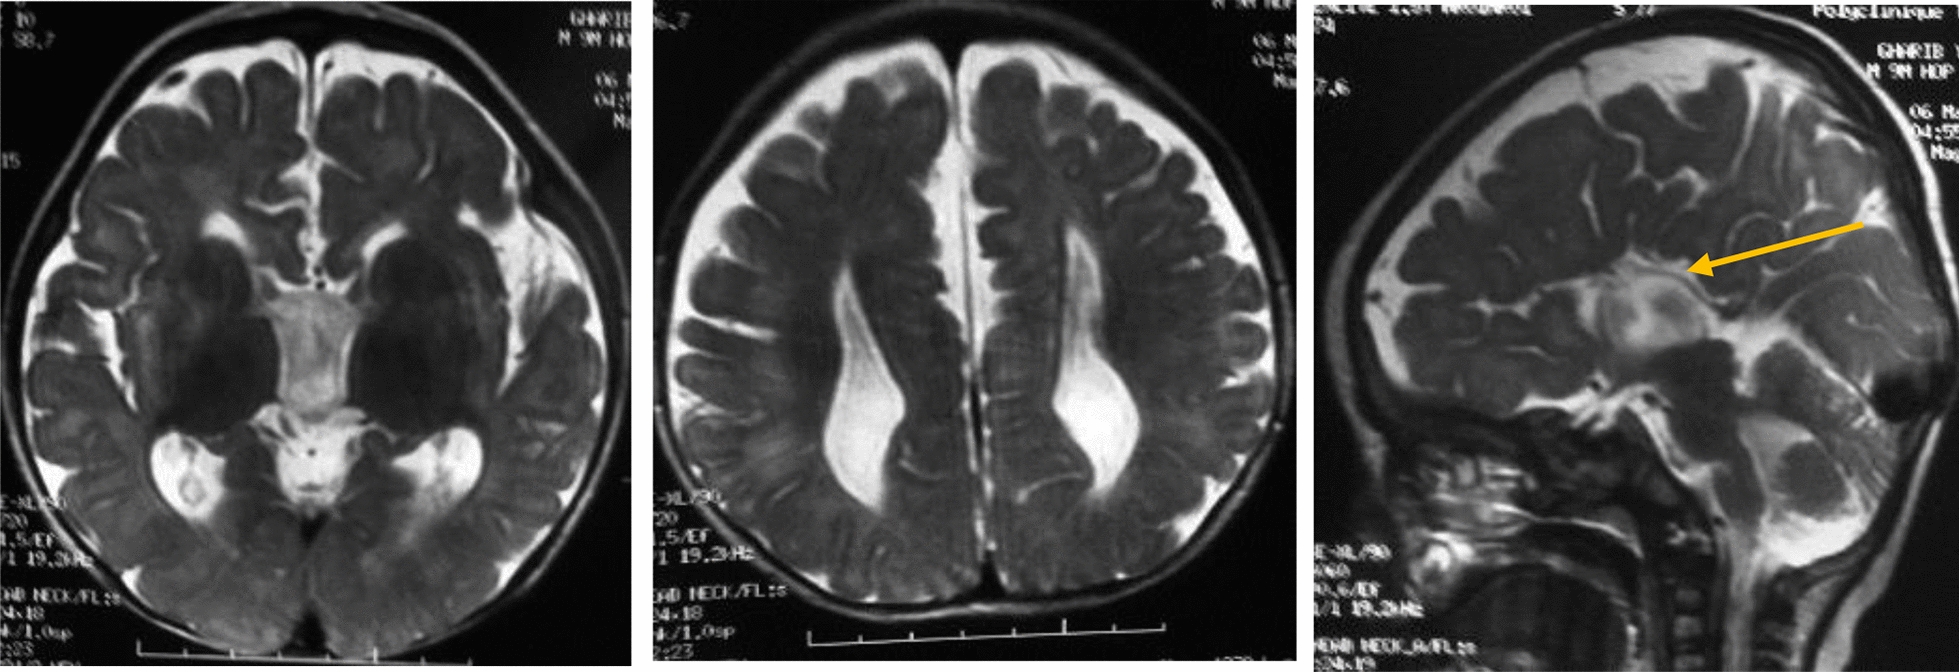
Patient 1 brain MRI showing*:* total callosal agenesis (yellow arrow) and brain atrophy associated with delayed encephalic myelination
